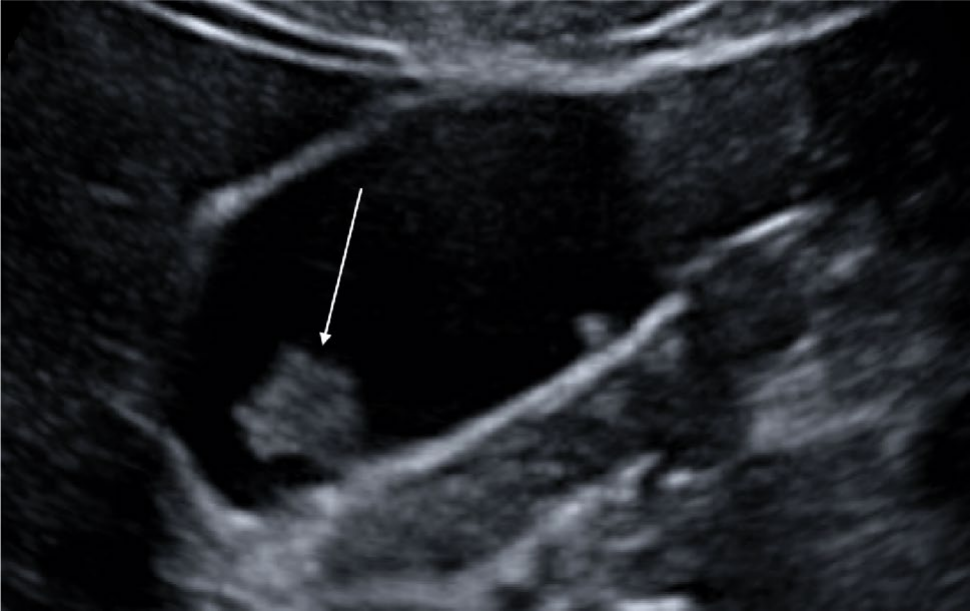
The sonographic image shows an inflammatory polyp (white arrow) that appears with a higher echogenicity than the liver parenchyma, homogeneous, pedunculated, and less than 10 mm in diameter
